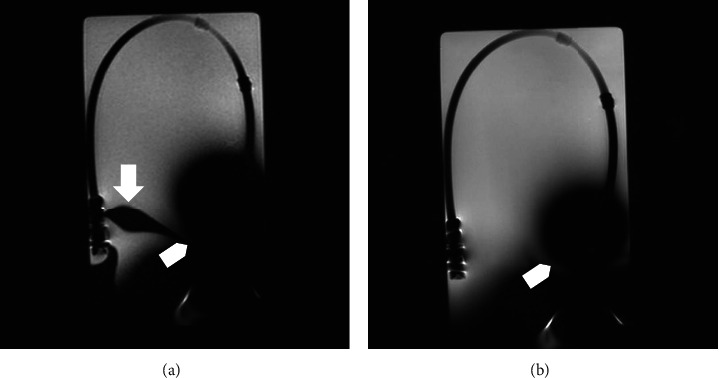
(a) Coronal fast gradient-echo T1-weighted and (b) coronal fast spin-echo T2-weighted images that demonstrate the extensive paramagnetic artifact with the “signal hole” of the tip tungsten ballast (arrowhead), the minor artifacts of the titanium connector (arrow), and the good visibility of the catheter.
